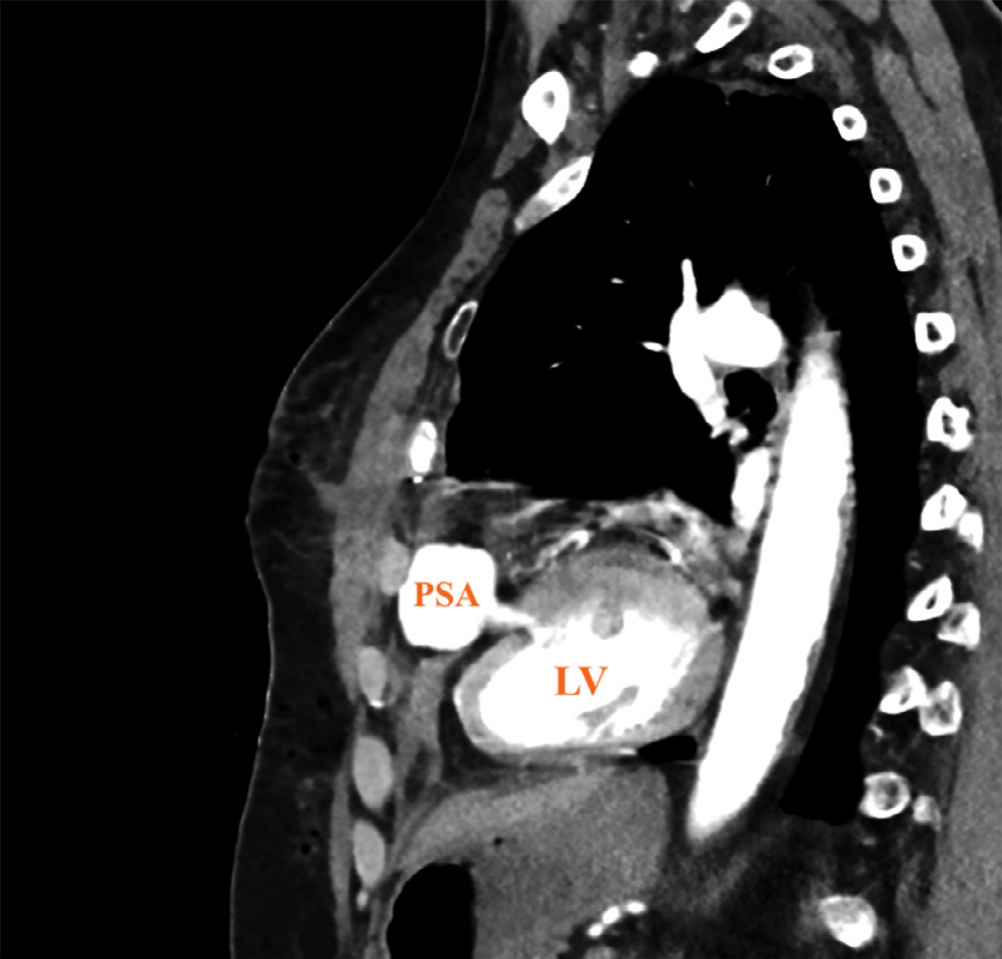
Sagittal Chest CT emphasizing the penetrating trajectory of the knife, coursing posteriorly above the left fifth rib and through the anterior wall of the left ventricle (LV). The penetrating wound traveling from the anterior chest wall to the left ventricle measured 10 cm in length. The large ventricular pseudoaneurysm (PSA) communicates with the left ventricle (LV).
